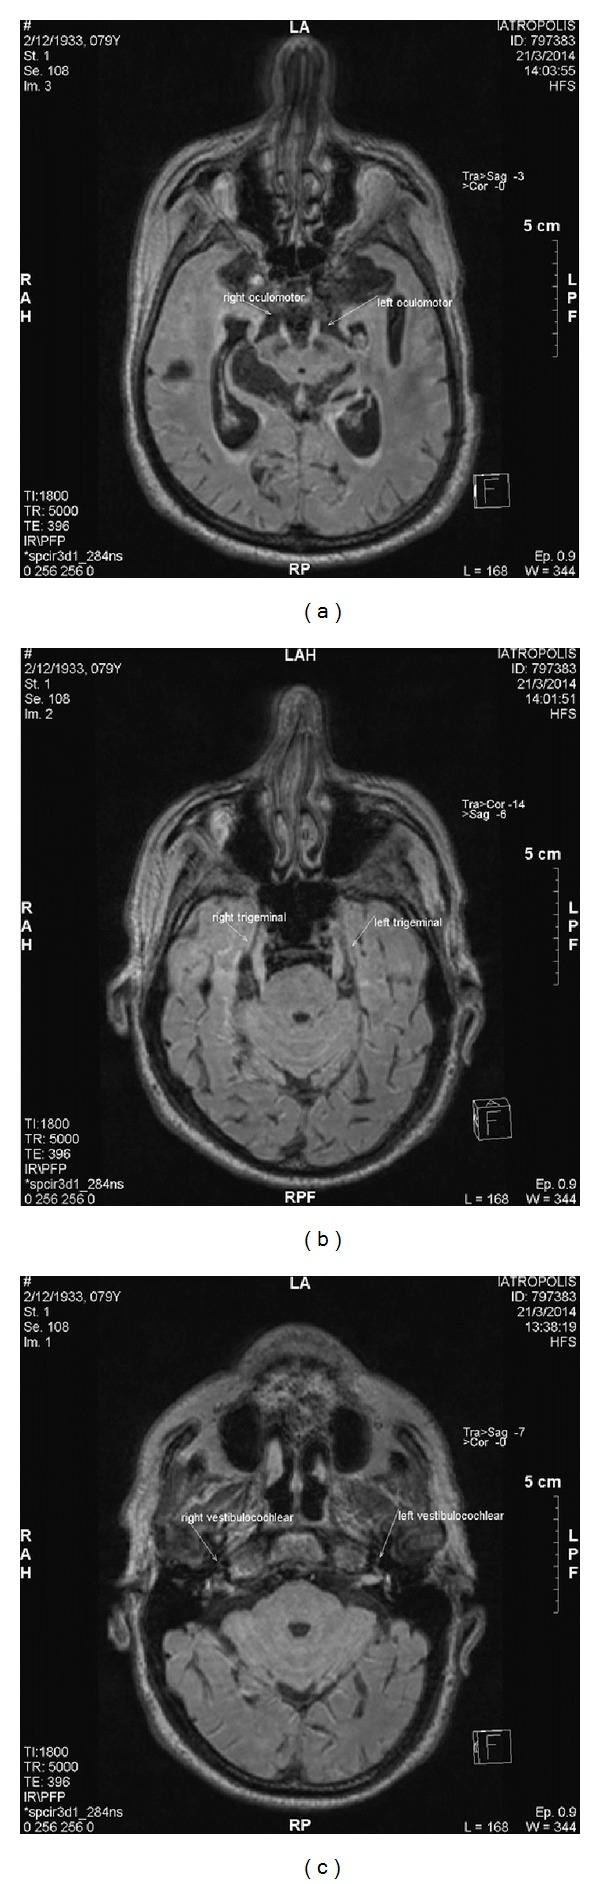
The MRI of the brain showed enhancement of the left trigeminal (a) and both oculomotor (b) and vestibulocochlear nerves (c) and signs of leptomeningeal disease.
